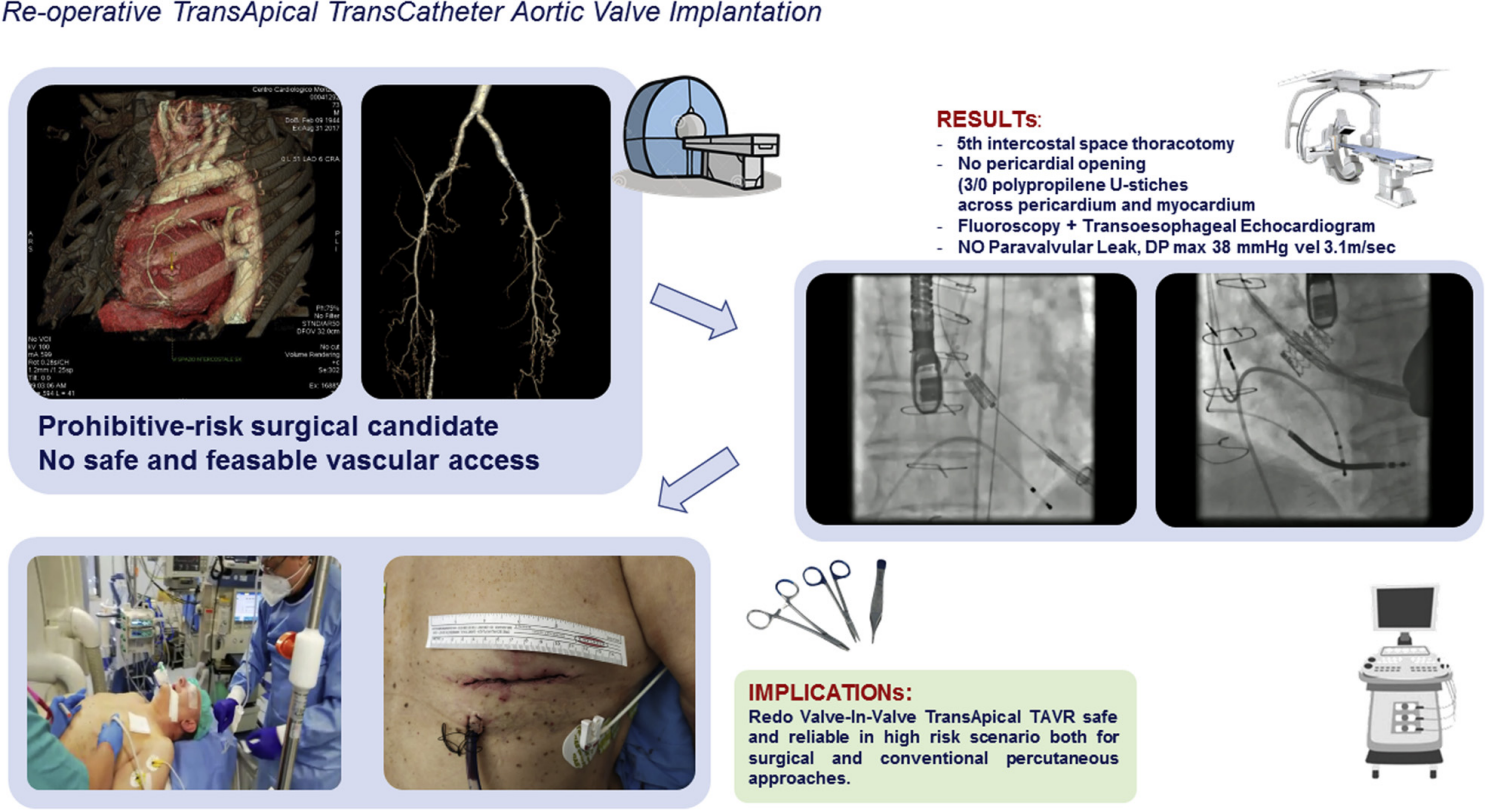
Brief description of the case and its management: computed tomography scan, catheter lab images, operating room setting, and skin wound at the end of the procedure.
